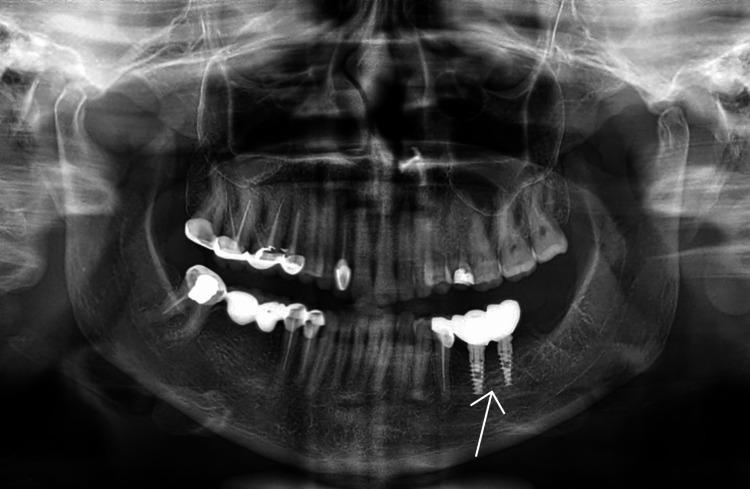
Postoperative OPG OPG: orthopantomagram
